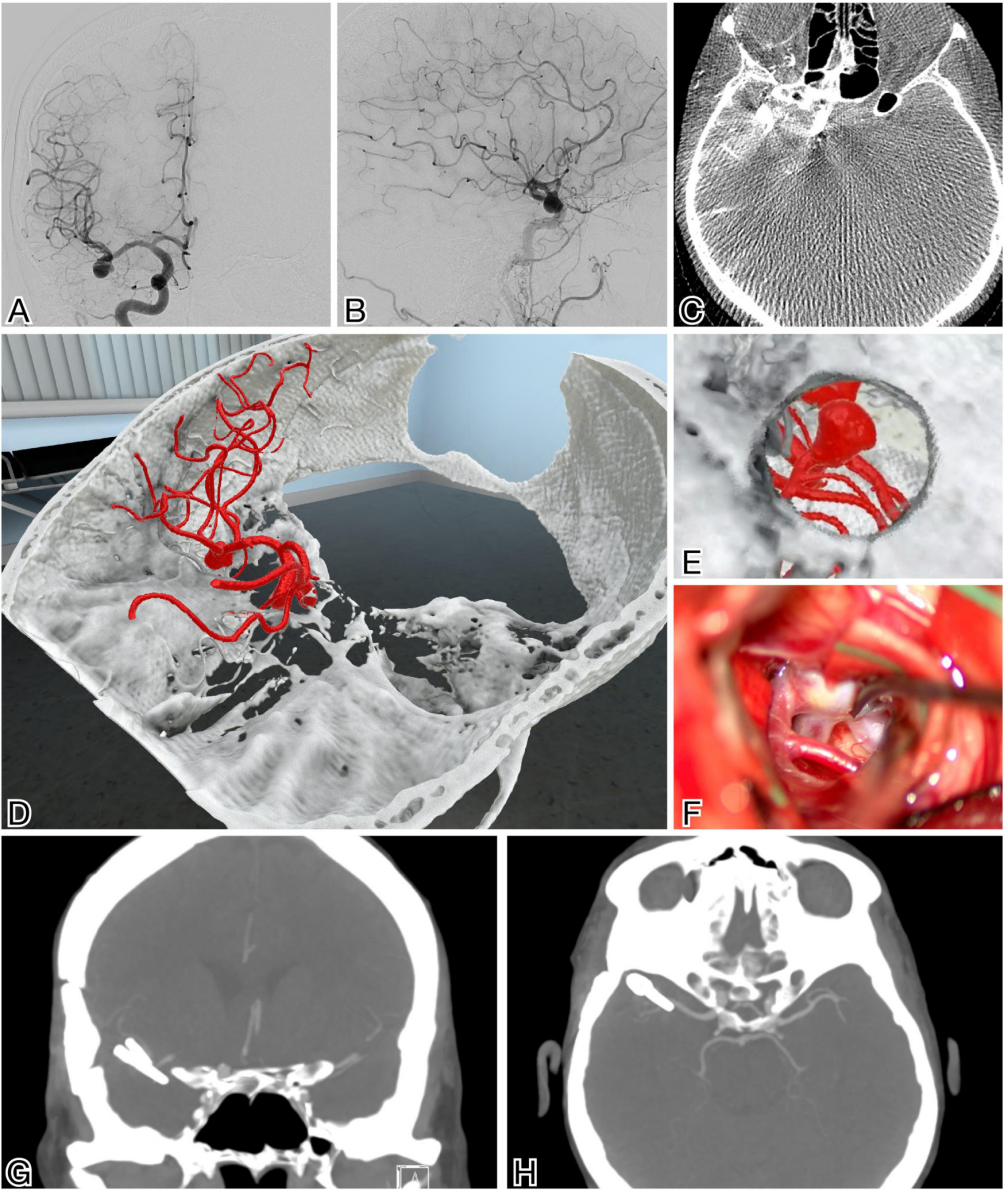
MCA aneurysm planning using VR as an adjunct for surgical planning. (A,B) Frontal and lateral views of the MCA aneurysm in DSA. (C) Dyna-CT with an arterial injection of the right internal carotid artery is used as input data for the VR reconstruction (D). Visualization of the aneurysm through the planned customized approach (E) in Specto Medical®. (F) Intraoperative view of the aneurysm with some of its anatomy covered and preoperatively analyzed in VR. (G,H) Postoperative CTA showing complete occlusion of the aneurysm.
